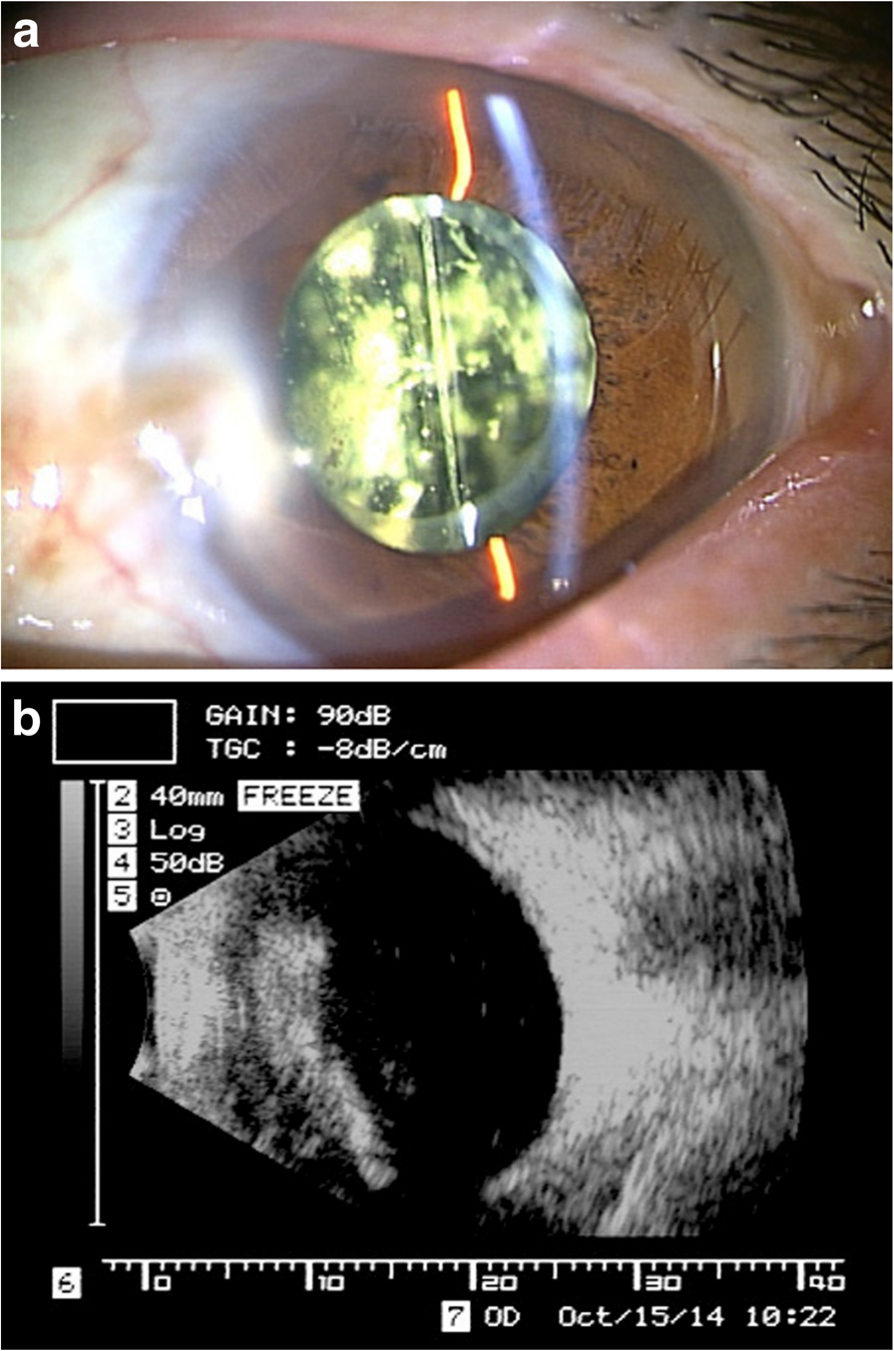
Ocular findings in a patient with asteroid hyalosis (AH) after successful cataract surgery. The asteroid bodies (ABs) appear to be concentrated in the anterior vitreous cavity of the left eye (a), and an ultrasonic B-mode image shows a shadow that appears to be a cluster of asteroid bodies (ABs) in the anterior vitreous cavity (b)
